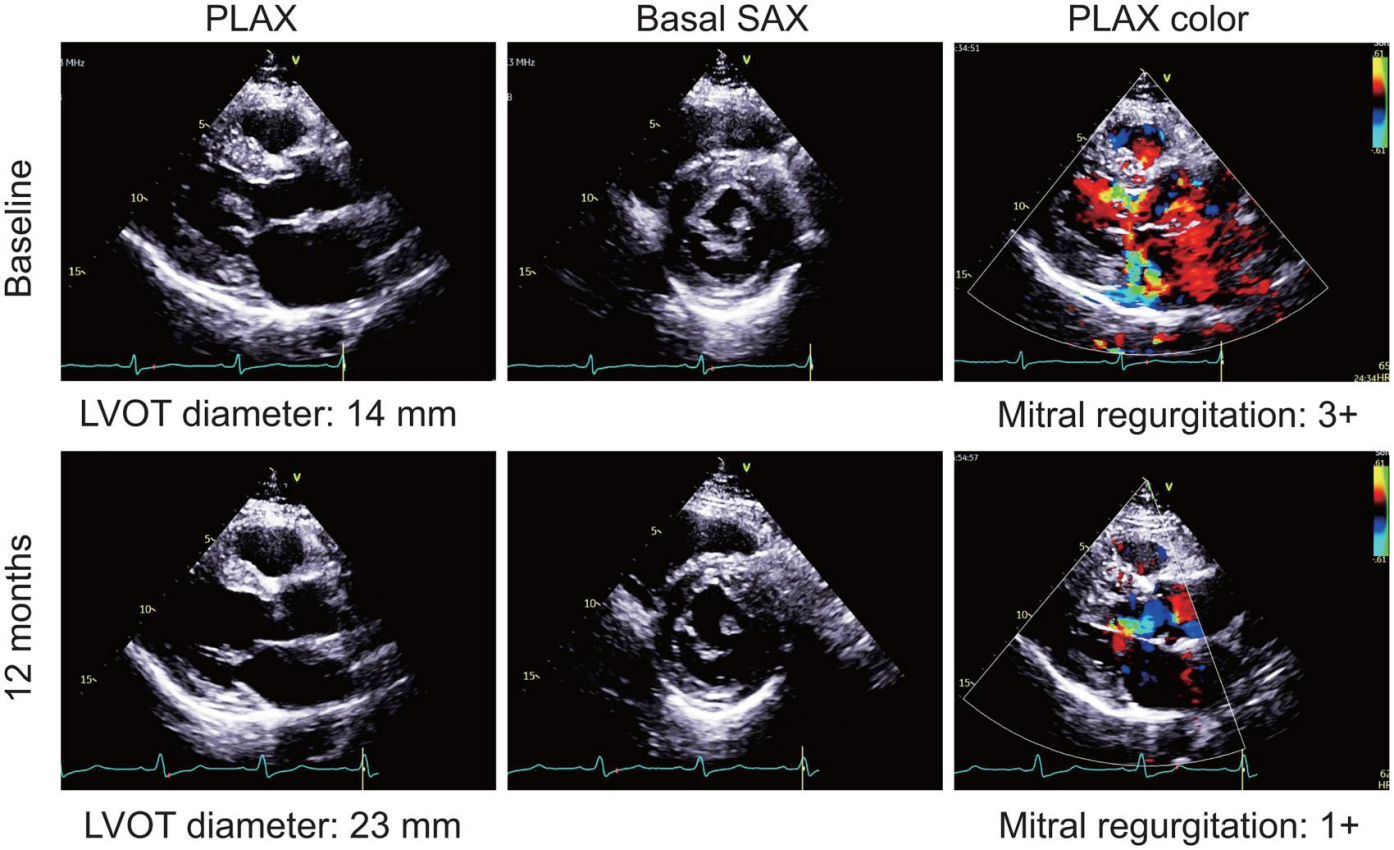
Representative Transthoracic Echocardiographic Images After Transapical Beating-Heart Septal Myectomy. The echocardiographic images showing the morphology and MR at baseline (top) and 12 months (bottom) after TA-BSM. Abbreviations: PLAX: parasternal long-axis view; SAX: short-axis view
